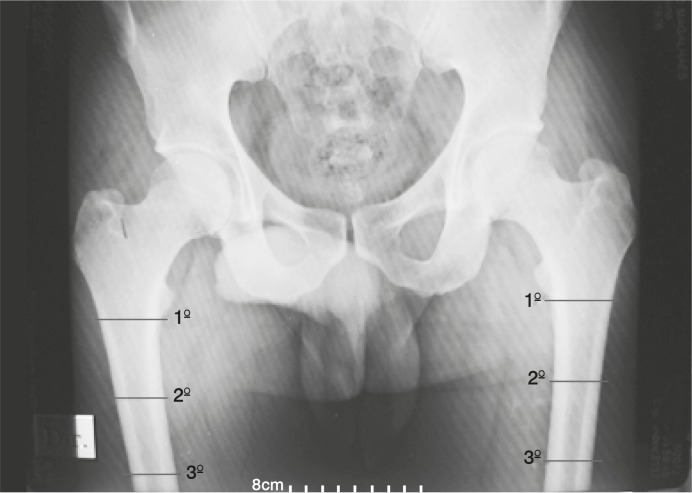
X-Ray showing the locations of the measurements performed.
